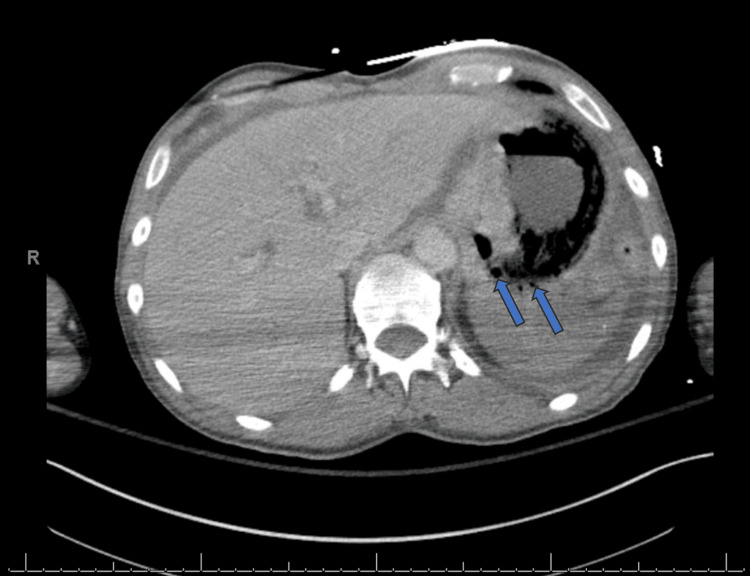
Computed tomography of the abdomen and pelvis showing emphysematous gastritis (arrows).
